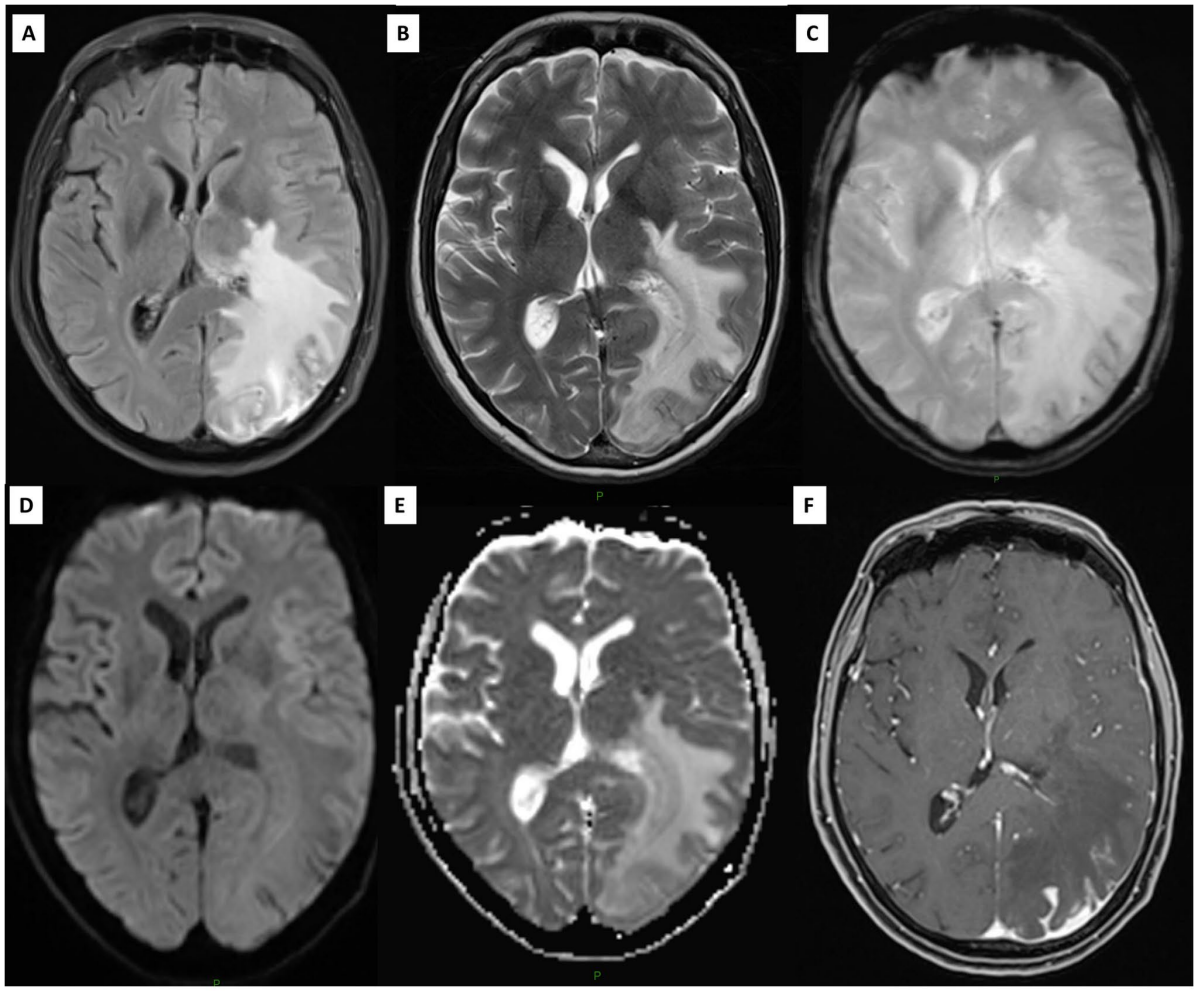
Brain MRI showing a pseudotumoral lesion in the left hemisphere. (A) Axial FLAIR and (B) T2-weighted imaging. (C) T2*-weighted imaging demonstrates a small intralesional hemorrhage (D,E) DWI and ADC confirm vasogenic edema of the lesion. (F) After gadolinium injection, parieto-occipital cortico-pial contrast enhancement is observed on the left side, with corresponding pachymeningeal thickening.
